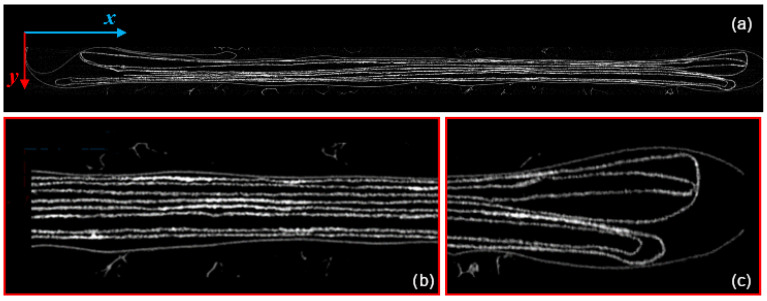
CT reconstruction of the document on the x/y plane. (a) The whole slice. (b) Zoom of the central area of the slices where the brightest pixels correspond to the ink (writings), the light gray-levels to the pages and the darker ones to the background. (c) Detail of the folded area.
